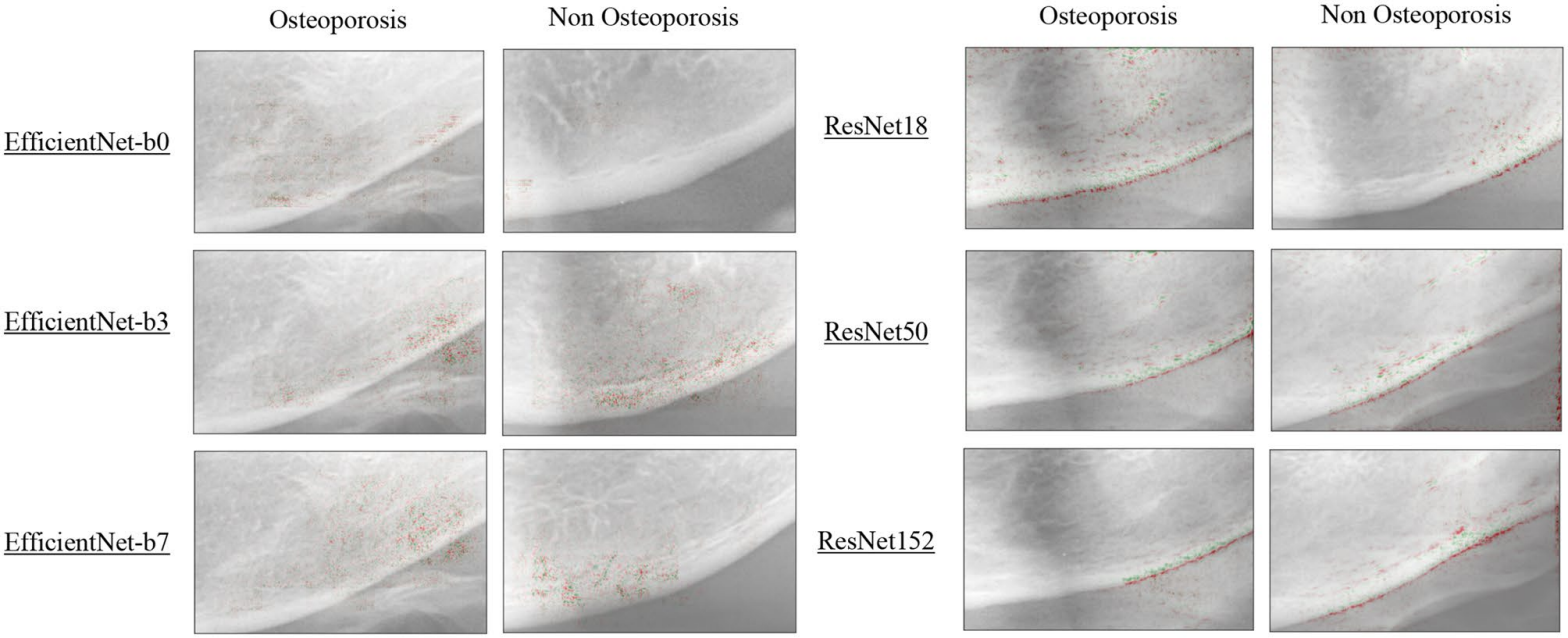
Visualization of characteristic regions of radiographs of osteoporosis and non-osteoporosis patient images using ResNet and EfficientNet.
